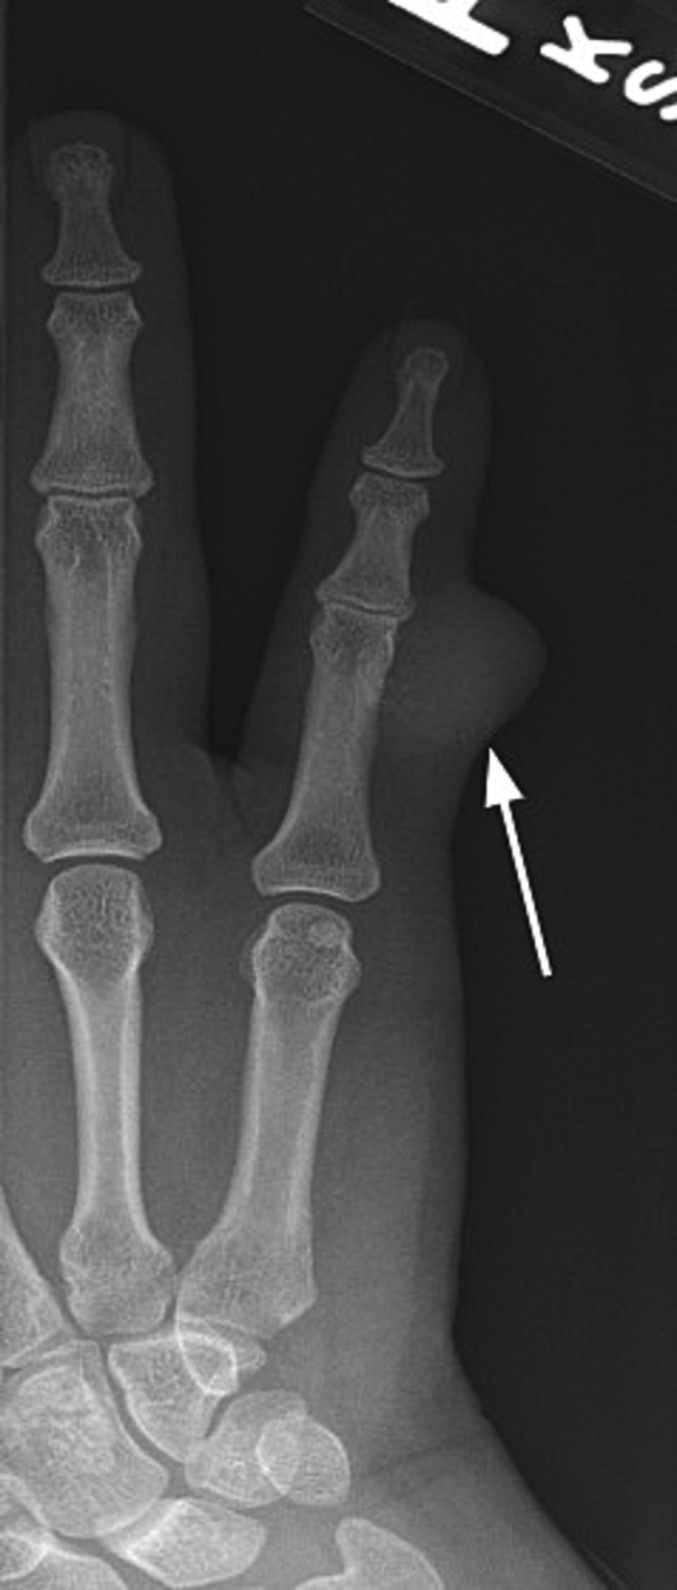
right finger x-ray showing the soft tissue mass and small calcifications present in the small finger of the patient, March 2022.
